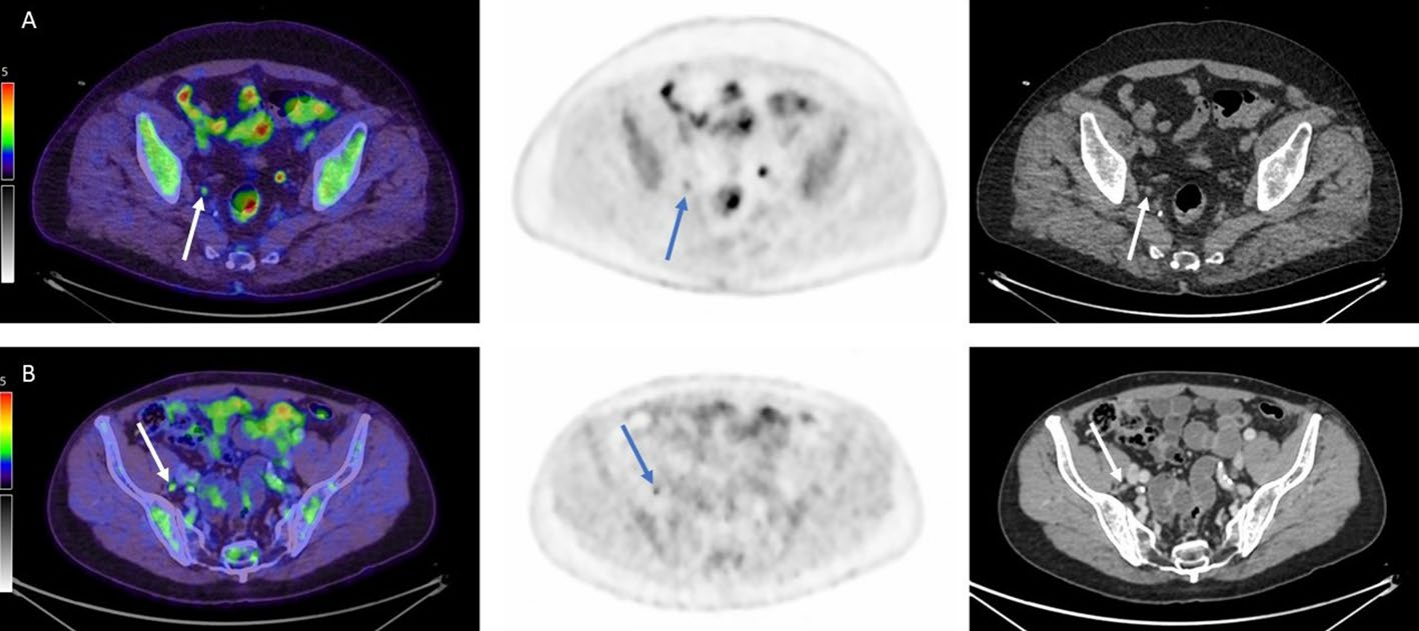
Patients with a true-positive (A) and false-positive (B) PET/CT scan, respectively. The arrows show small lymph nodes regarded as suspected lymph node metastases on PET/CT. Fused PET/CT images (left), PET images (middle) and CT images (right)
